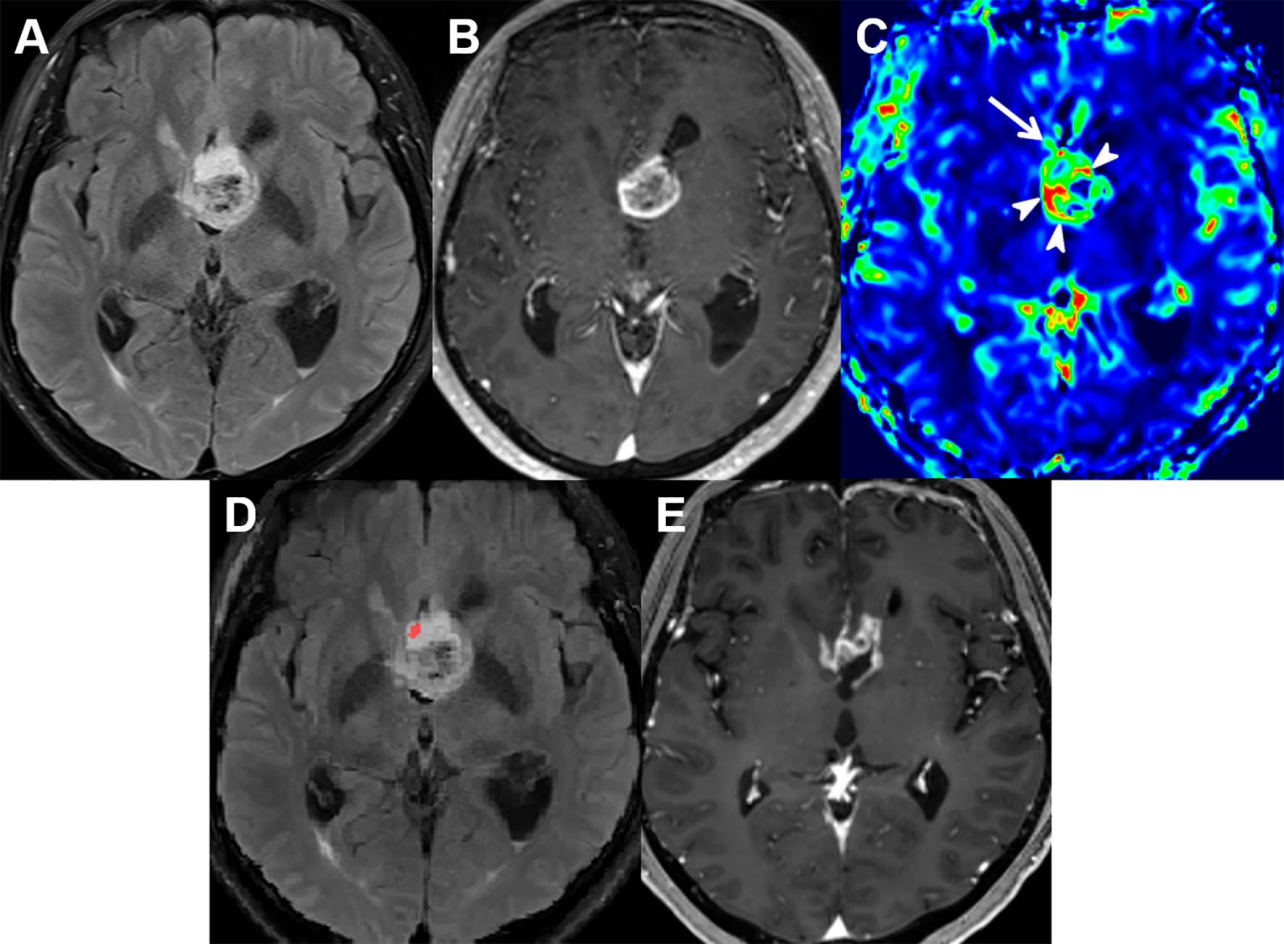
A 58-year-old woman with glioblastoma in the training set. (A,B) On axial preoperative T2 FLAIR and CE T1W images, a heterogeneously enhancing mass was found at the septum pellucidum and corpus callosum along with a small surrounding area of non-enhancing T2 hyperintensity. (C) nCBV is mildly increased at the anterior aspect (arrow) of the enhancing tumor, which shows an overt increase in nCBV (arrowheads). (D) The multiparametric MRI model predicted early local progression to occur at the red area anterior to the enhancing tumor. (E) Follow-up MR images at 7 months confirmed local progression at the corpus callosum. *FLAIR* fluid-attenuated inversion recovery, *CE T1W* contrast-enhanced T1-weighted.
